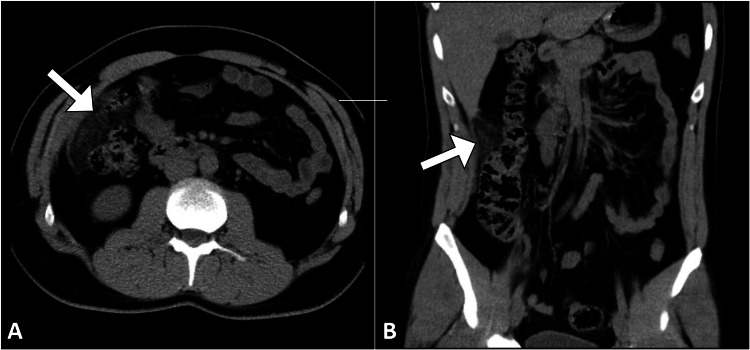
Axial (A) and coronal (B) CT images display a localized focal area of increased fat attenuation within the omentum (arrow), consistent with acute omental infarction. CT: computed tomography.
